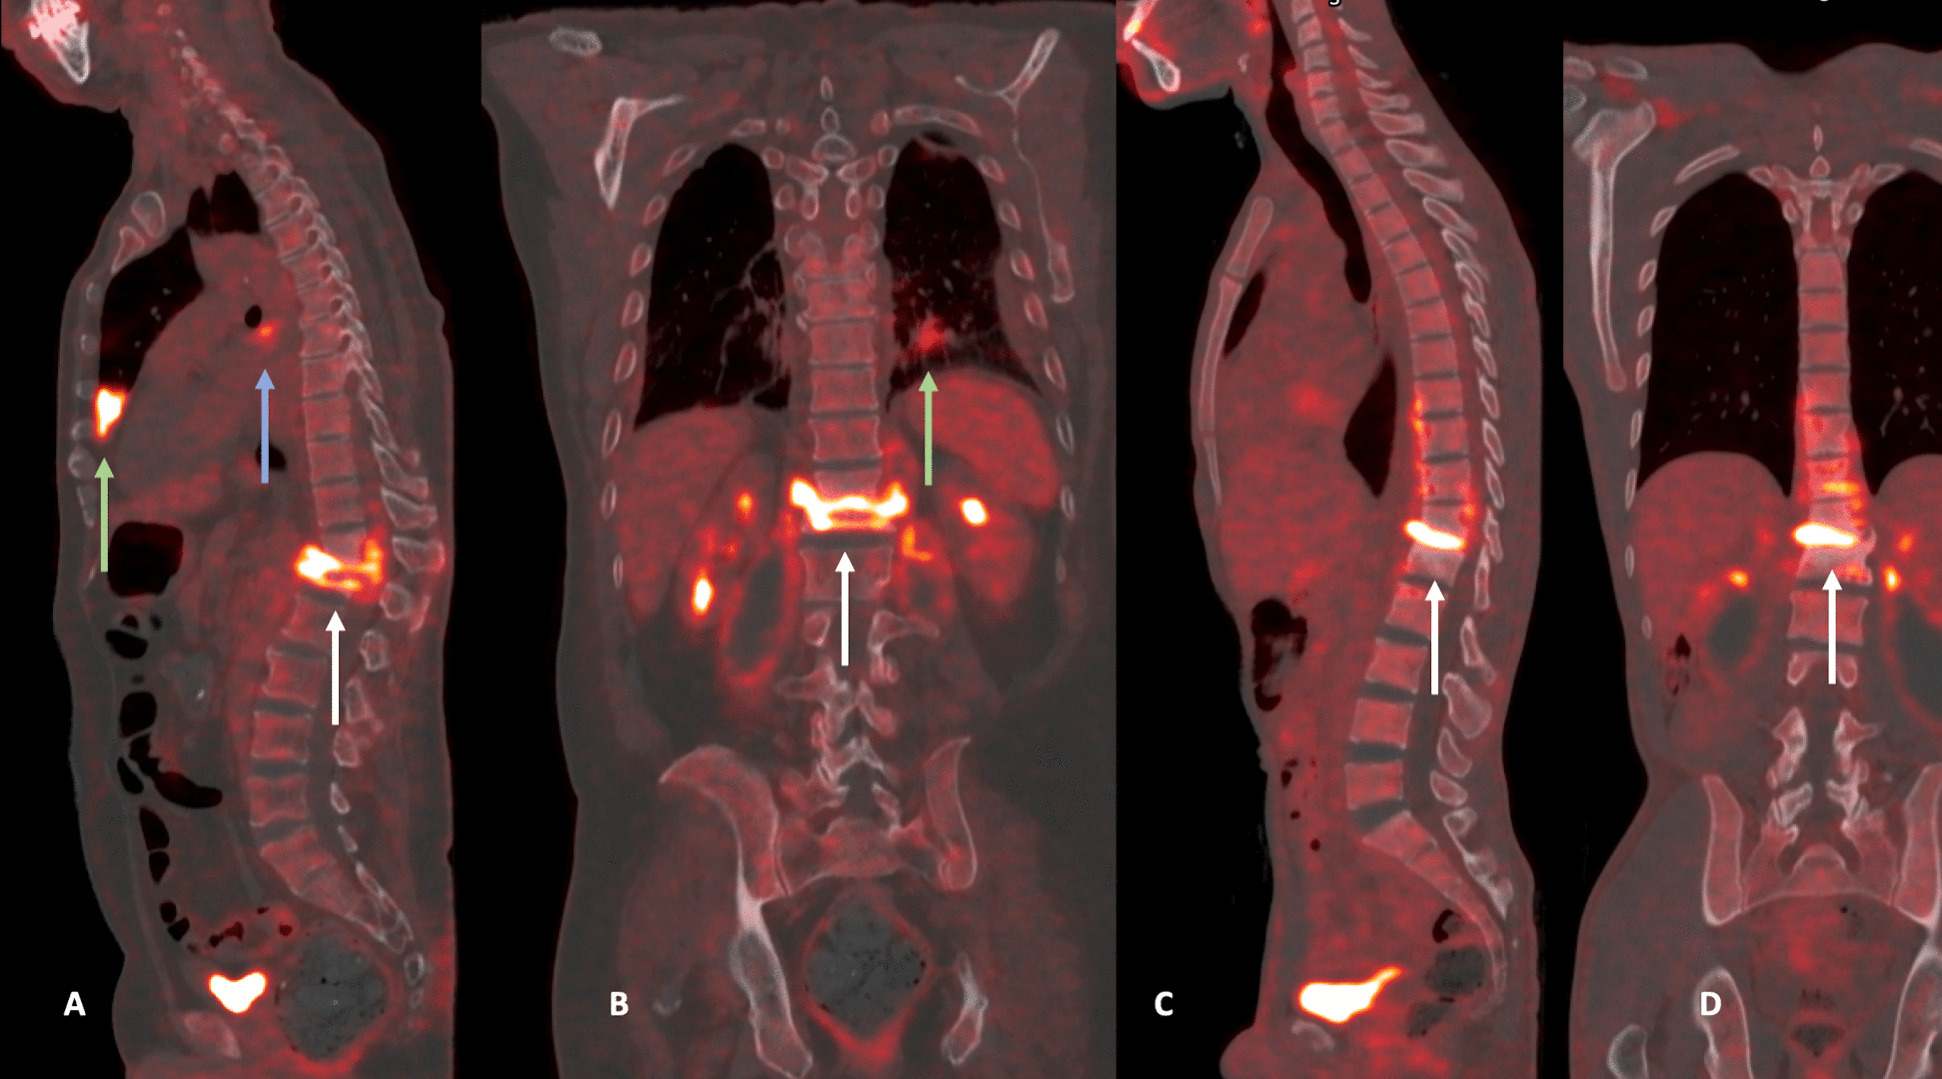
Example ^18^FDG-PET/CTs of two patients with spinal tuberculosis (TB). Patient 1 with sagittal (A) and coronal plane (B) with disseminated spinal tuberculosis. Spinal TB [white arrow] with a pulmonary TB lesion [green arrow] and mediastinal lymph node with FDG uptake [blue arrow]. Patient 2 with sagittal (C) and coronal plane (D) with isolated spinal tuberculosis. Spinal TB [white arrow] with no other lesion in the body in ^18^FDG-PET/CT
